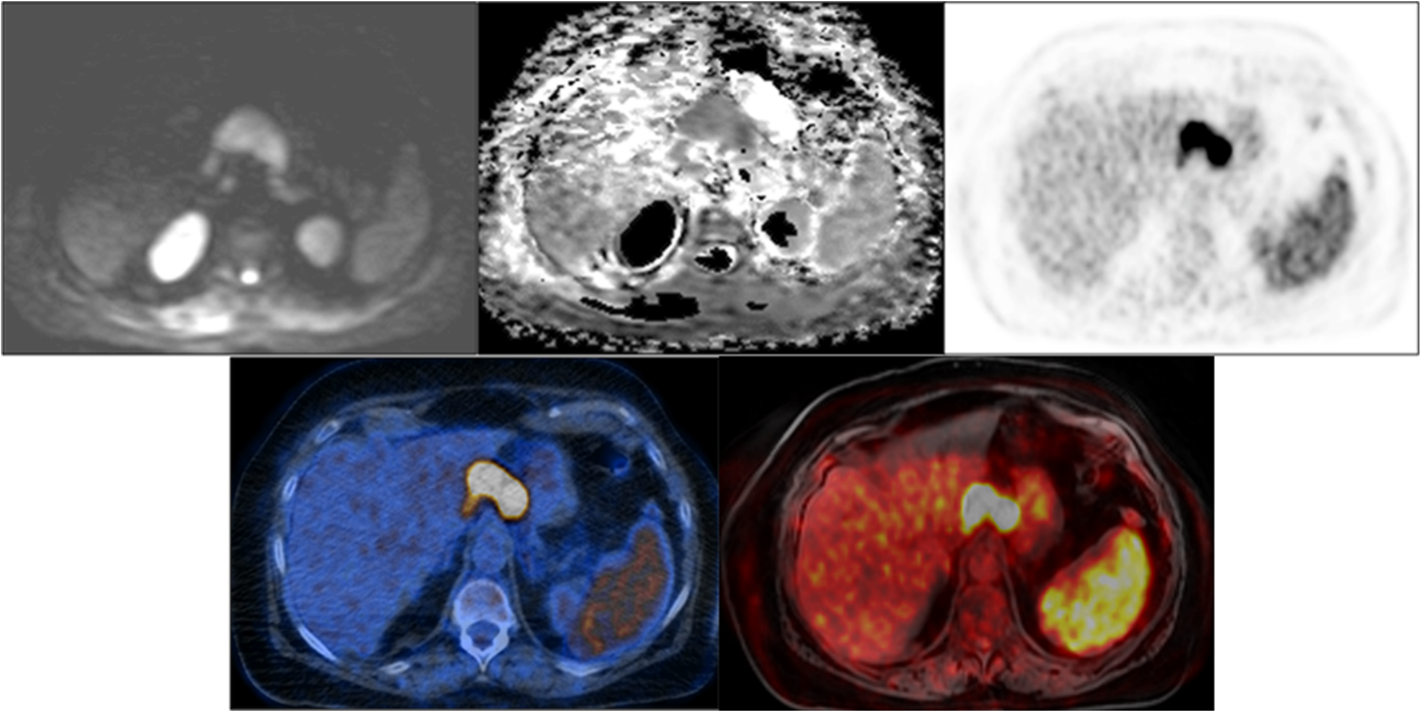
A female patient with a diffuse large B-cell lymphoma stage IVB. PET/CT/MRI for initial staging. Top: Axial WB-DW-MRI (b value = 800) and axial ADC map show restricted diffusion in a lymph node conglomerate in the upper abdomen (calculated ADC_mean_ = 0.72 × 10^−3^ mm^2^/s), but no restricted diffusion in the spleen (calculated ADC_mean_ = 1.37 × 10^−3^ mm^2^/s). Axial PET shows uptake in the same lymph node conglomerate but also diffuse uptake in the spleen, which was significantly higher than liver uptake. Bottom: FDG-PET/CT and FDG-PET/MRI show FDG avidity in both the lymph node mass and the spleen, indicating lymphoma manifestation
